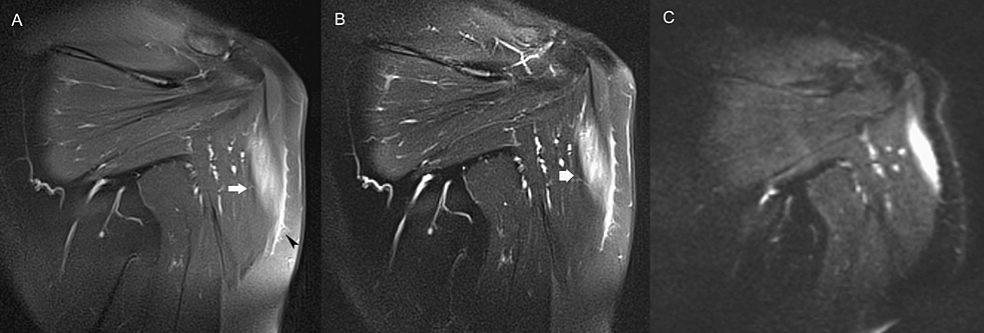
COVID-19 vaccine-related myositis in a middle-aged woman. (A) Coronal T2-weighted MR image with fat saturation shows edema infiltrating the deltoid muscle (arrow). Note the perifascial fluid (arrowhead). (B) Coronal T1-weighted MR image with fat saturation after gadolinium administration shows intense enhancement of inflamed deltoid muscle (thick arrow). (C) Coronal diffusion-weighted image (b-value 800 s/mm^2^) shows increased diffusion in the deltoid muscle, representing increased capillary perfusion due to active inflammation.
